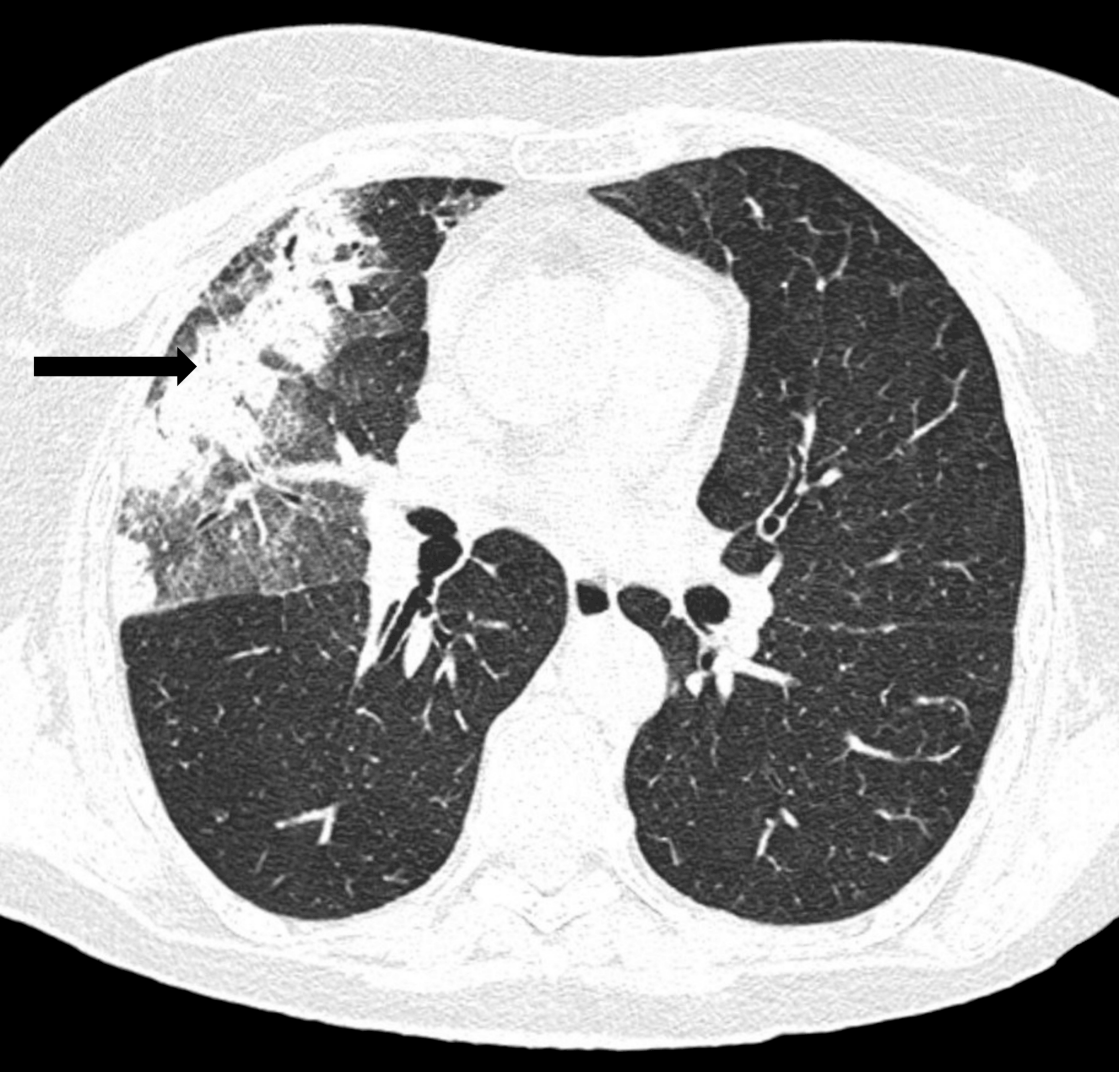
Thoracic CT scan revealing right parenchymal consolidation, permeated by air bronchograms. Parenchymal consolidation in the right lung, permeated by air bronchograms (black arrow).
